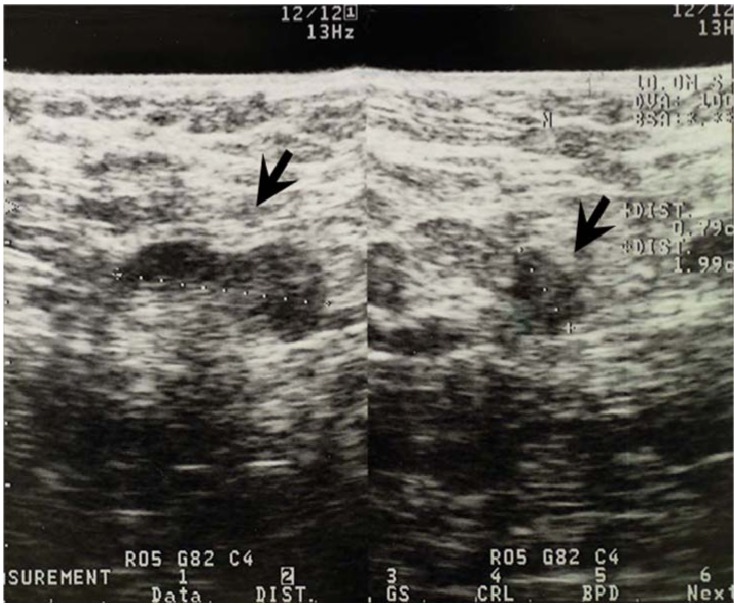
Ultrasonographic appearance of type 2 lymph node metastases. This lymph node (arrow) shows well-defined boundaries and weak internal echoes.
